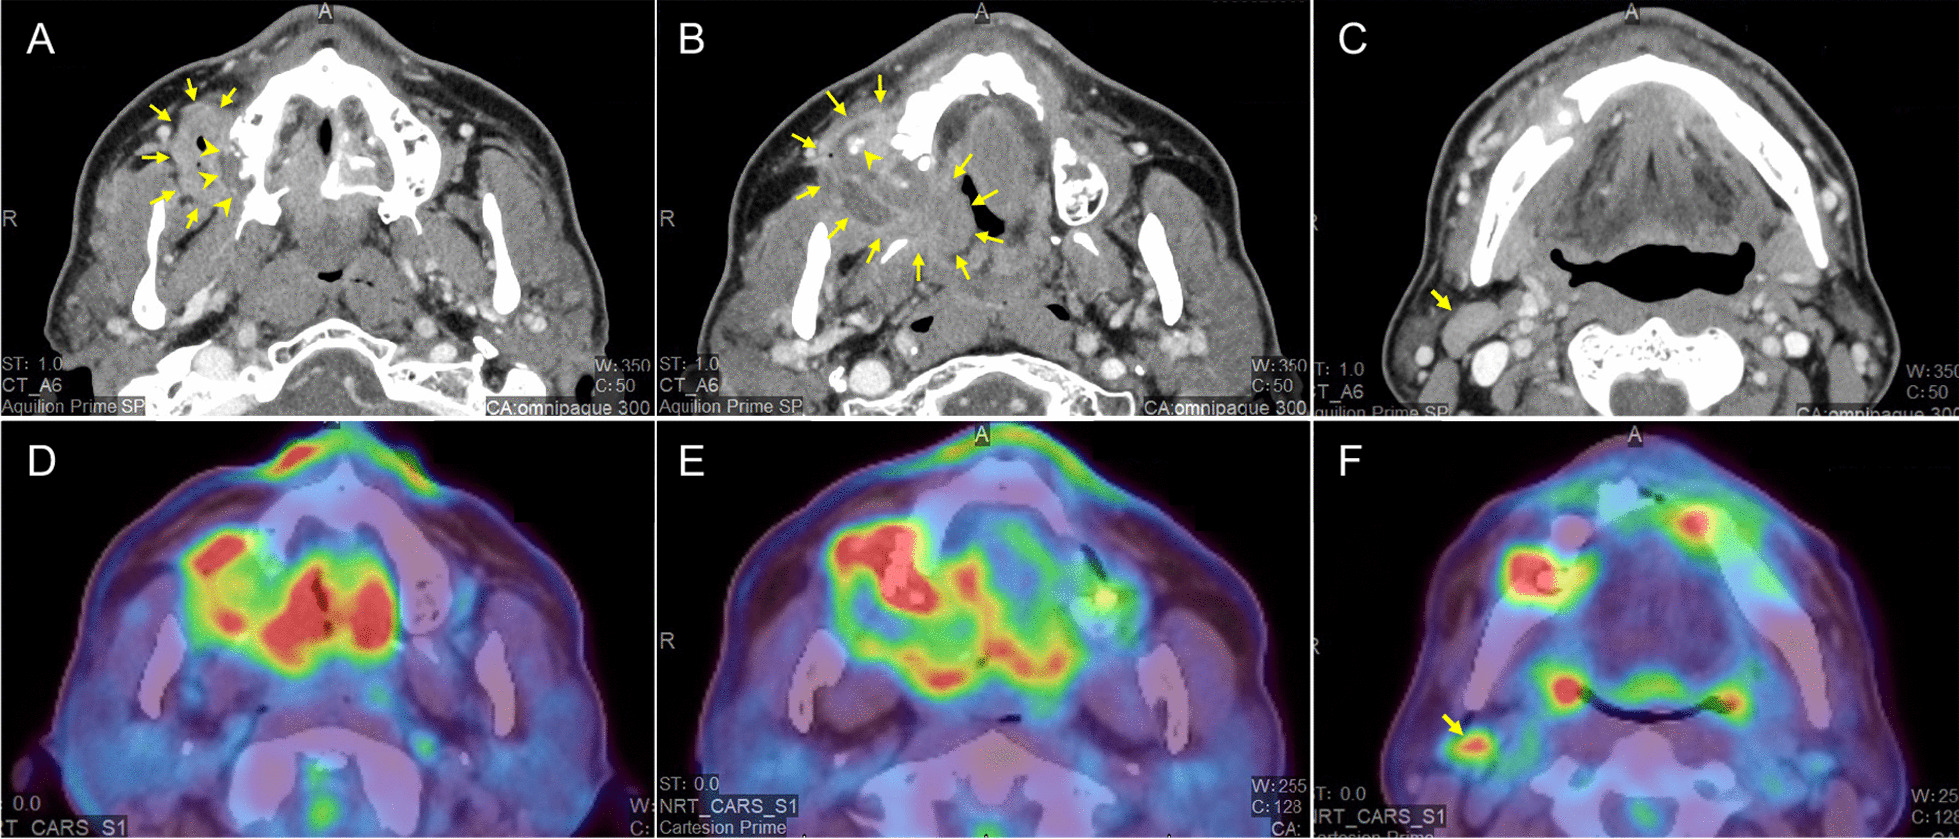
CT and PET/CT findings. A–C CT image showing a marked bone destruction-like defect on the lateral side of the right maxillary alveolus (arrowhead, A) contiguous with the right maxillary gingival mass lesion (arrows, A and B), along with small calcifications (arrowhead, B) anteriorly within the mass. Multiple enlarged lymph nodes, nearly 20 mm in length, were observed in the level I–II region of the right side of the neck (arrows, C). D–F PET/CT scan demonstrating FDG hyperaccumulation (SUVmax = 14.81) in the right maxilla in an area consistent with the gingival lesion containing chronic periodontitis (D and E). Only mild FDG accumulation was observed in the cervical lymph nodes (arrows, F)
